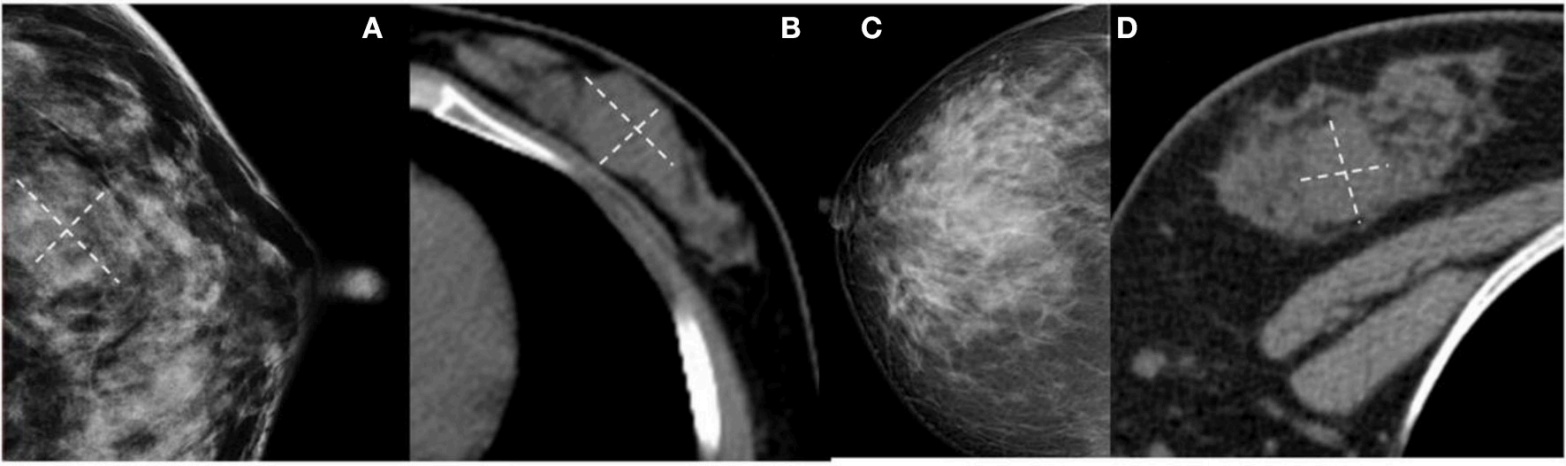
(A,B) Benign Phyllodes tumor (PT) of left breast in 55-year-old woman. Mammogram shows an ill-defined isodensity mass. However, CT can show the boundary clearly. (C,D) Benign PT of right breast in 34-year-old woman. The lesion is not visible on mammogram, but clearly visible on CT. They are all affected by the cover effect of mammography.
